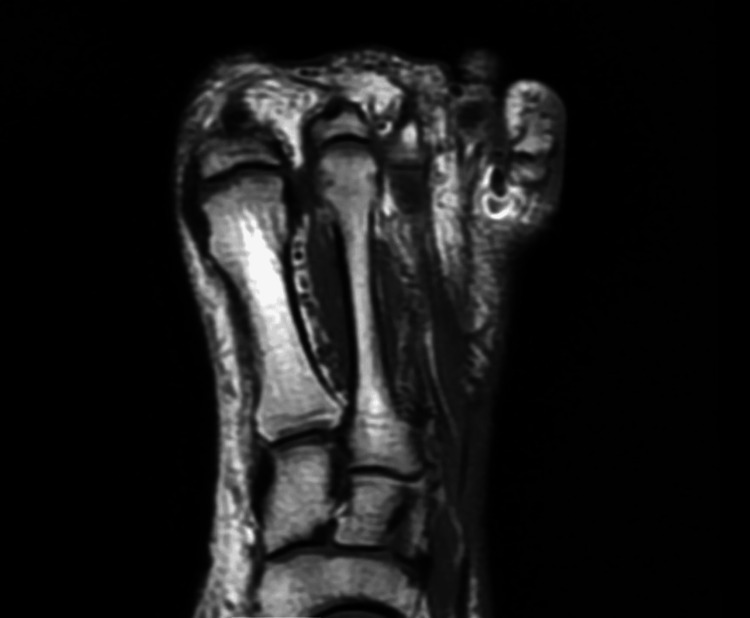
MRI of the left foot demonstrating soft-tissue edema. Coronal MRI of the left foot shows diffuse soft-tissue edema concerning for cellulitis. No evidence of abscess formation or osteomyelitis is identified.
